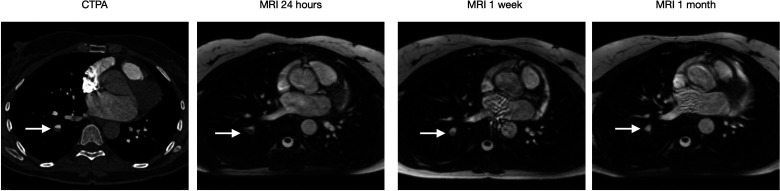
(Four images) A 49-year-old female visited ER after one week of dyspnoea and chest pain. CTPA showed PE (arrow) at the lower right lobe level. MRI shows a complete resolution at one month.
